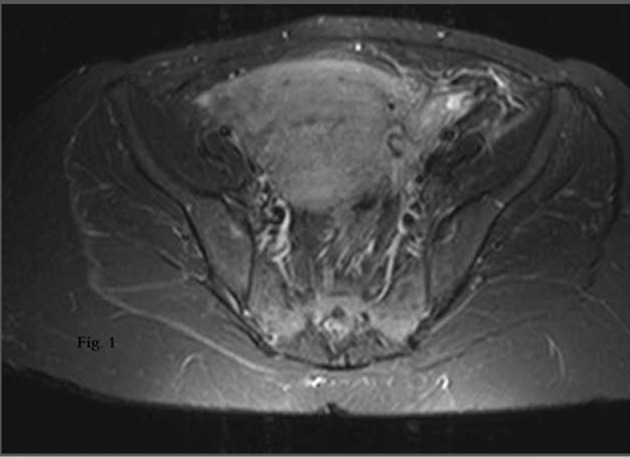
MRI of left lower myometrial part of the uterus: abruption with simultaneous presence of hemorrhagic stuff.
